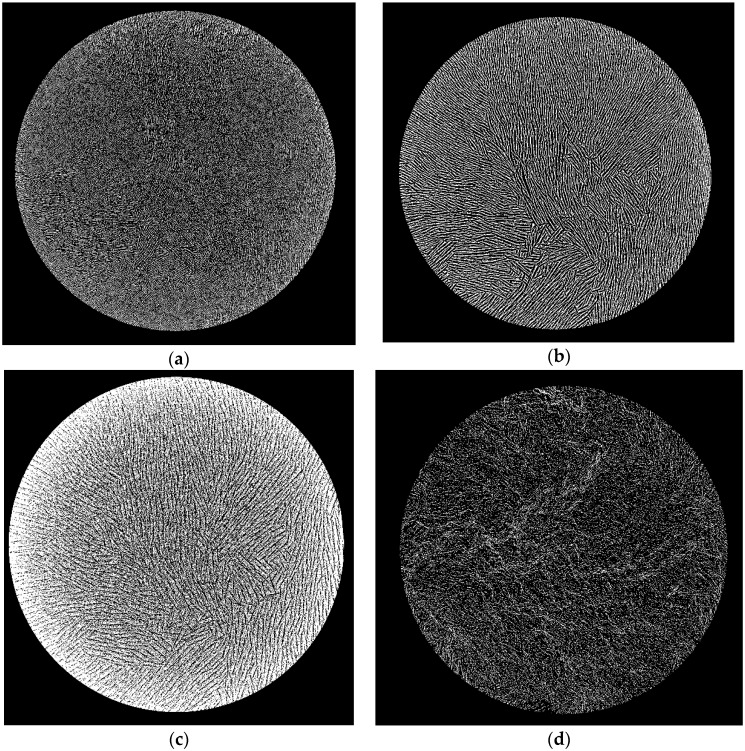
X-ray micro-computed tomography images of cross-sections through monoliths at different heights, h (cf. Figure 1a). (a) φ_v_ = 0.128, h = 0.90; (b) φ_v_ = 0.128, h = 0.5; (c) φ_v_ = 0.128, h = 0.18; (d) φ_v_ = 0.032, h = 0.9.
